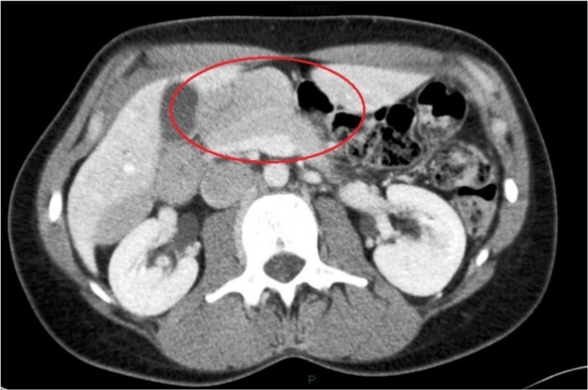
Computed tomography imaging of the intra-peritoneal duodenum (C-shaped portion on the red ring)
